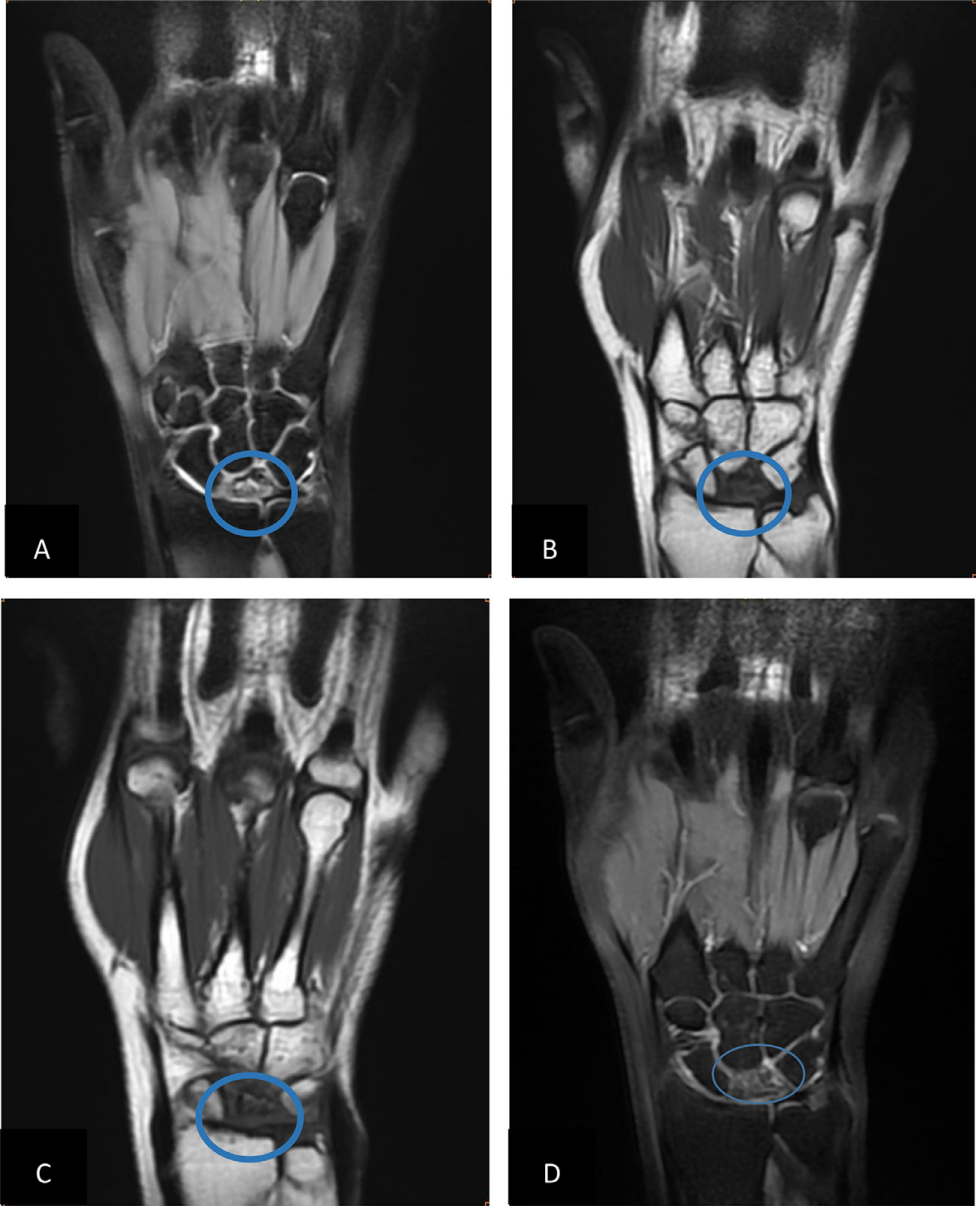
MRI of the right hand with coronal PD Fat Sat sequence (A), T1-weighted coronal sequence (B, C), and T1-weighted coronal sequence with fat suppression and gadolinium injection (D) revealed a collapsed lunate with homogeneous T1hyposignal and heterogeneous DP hypersignal, predominant in its anterior horn, slightly enhanced after contrast.
